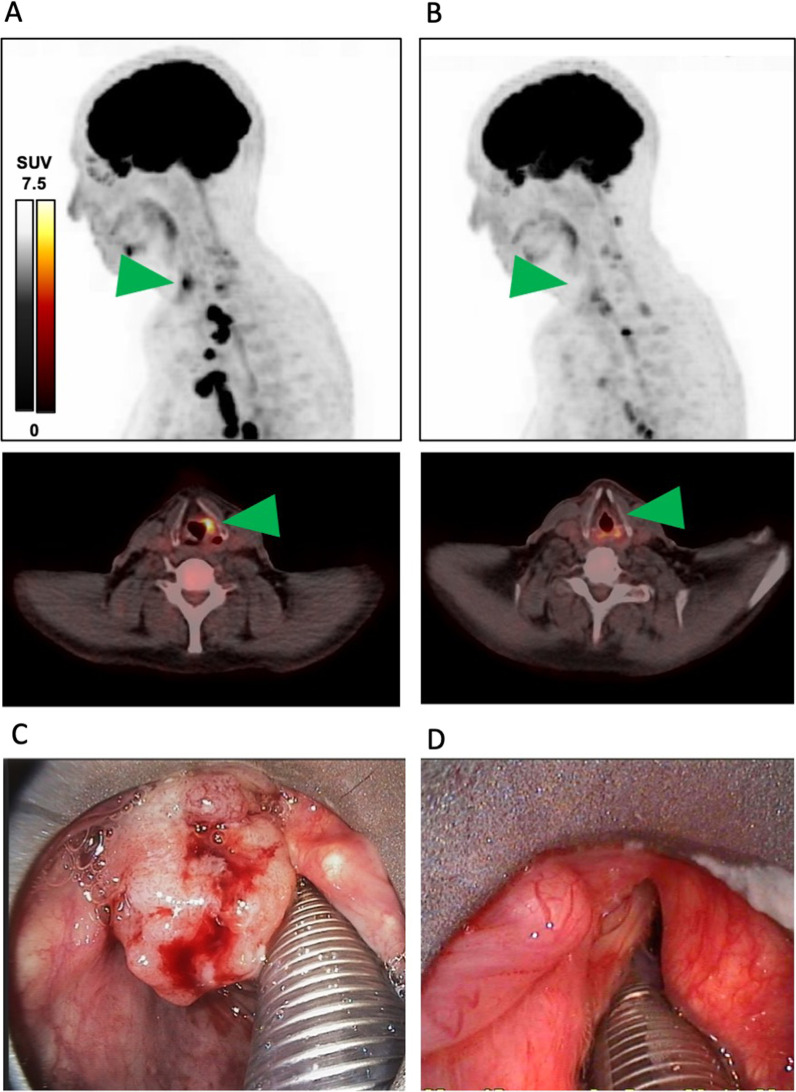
Therapy response after 4 cycles pembrolizumab/carboplatin/pemetrexed. Maximal intensity projection (MIP) of [^18^F]FDG PET/CT A before treatment start, B after 4 cycles immuno-chemotherapy. In A, B the area of the supraglottic laryngeal cancer is indicated by a green arrow. C Microlaryngoscopic view of the laryngeal cancer before treatment start during diagnostic panendoskopy. D Microlaryngoscopic view of the same area after 4 cycles immuno-chemotherapy during a control panendoscopy
